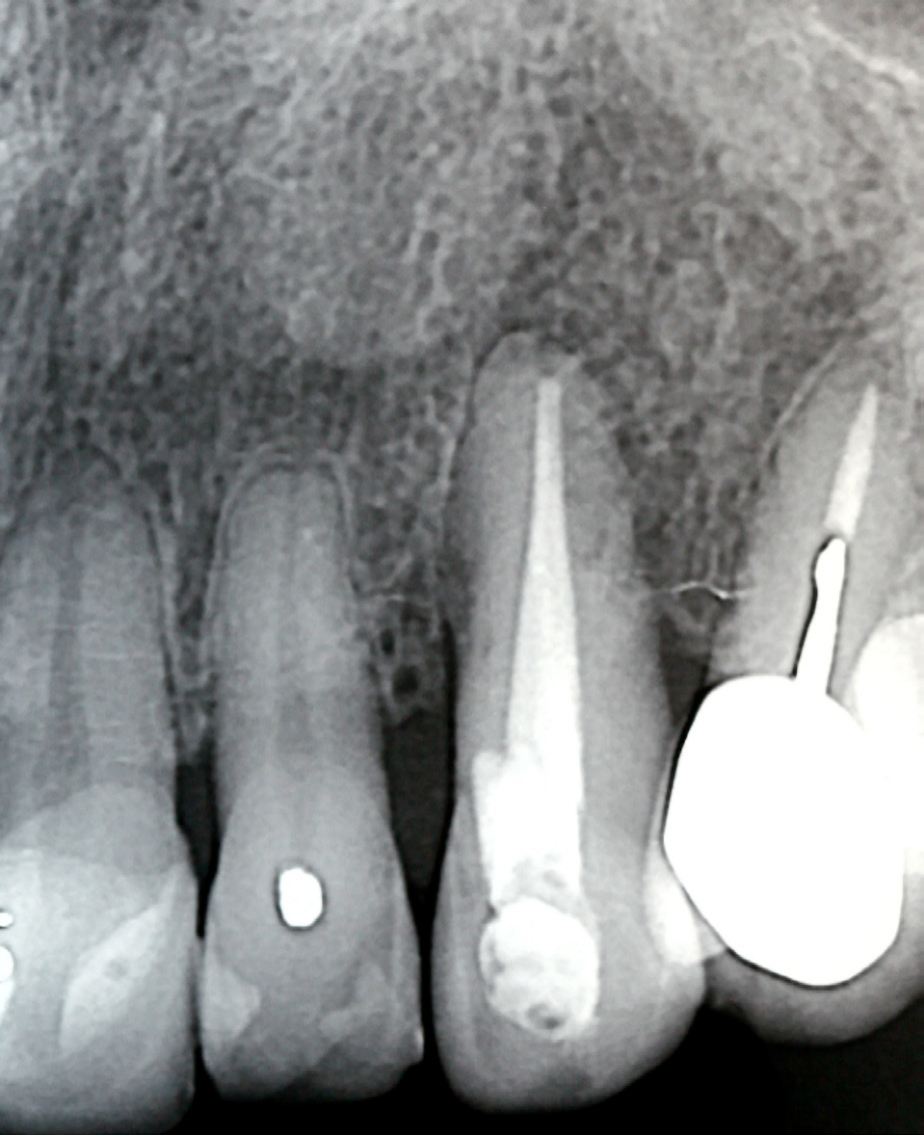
Radiograph shows a complete resolution of the lesion after 18 years.
